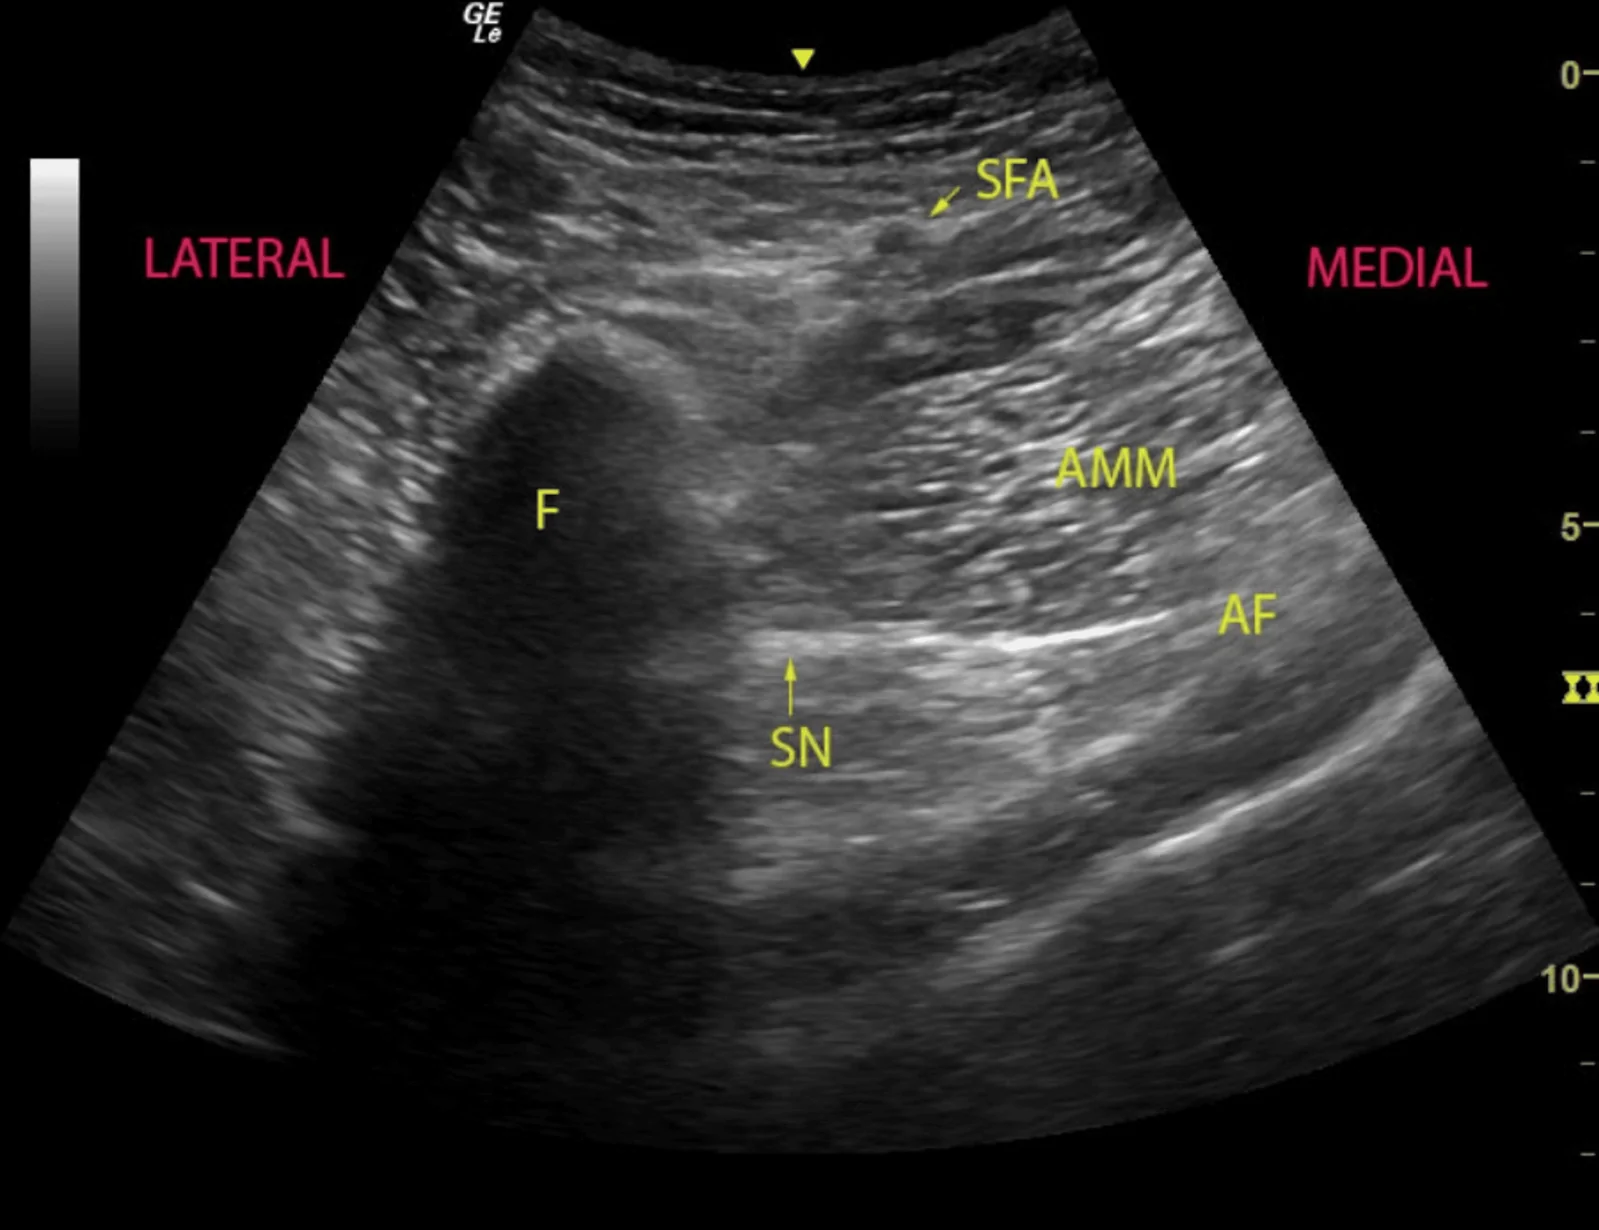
Ultrasound image before needle placement. The curved array transducer is placed in the proximal third of the thigh medial to the femur at the level of the proximal adductor canal block. The sciatic nerve (SN) is well visualized 2-3 cm medial to the femur (F) and inferior to the adductor fascia (AF).  Note the superficial femoral artery (SFA) which must be visualized prior to needle advancement. AMM, adductor magnus muscle Source: Halpern et al., 2022 [6]; reproduced with permission from Taylor & Francis Informa UK Ltd.
